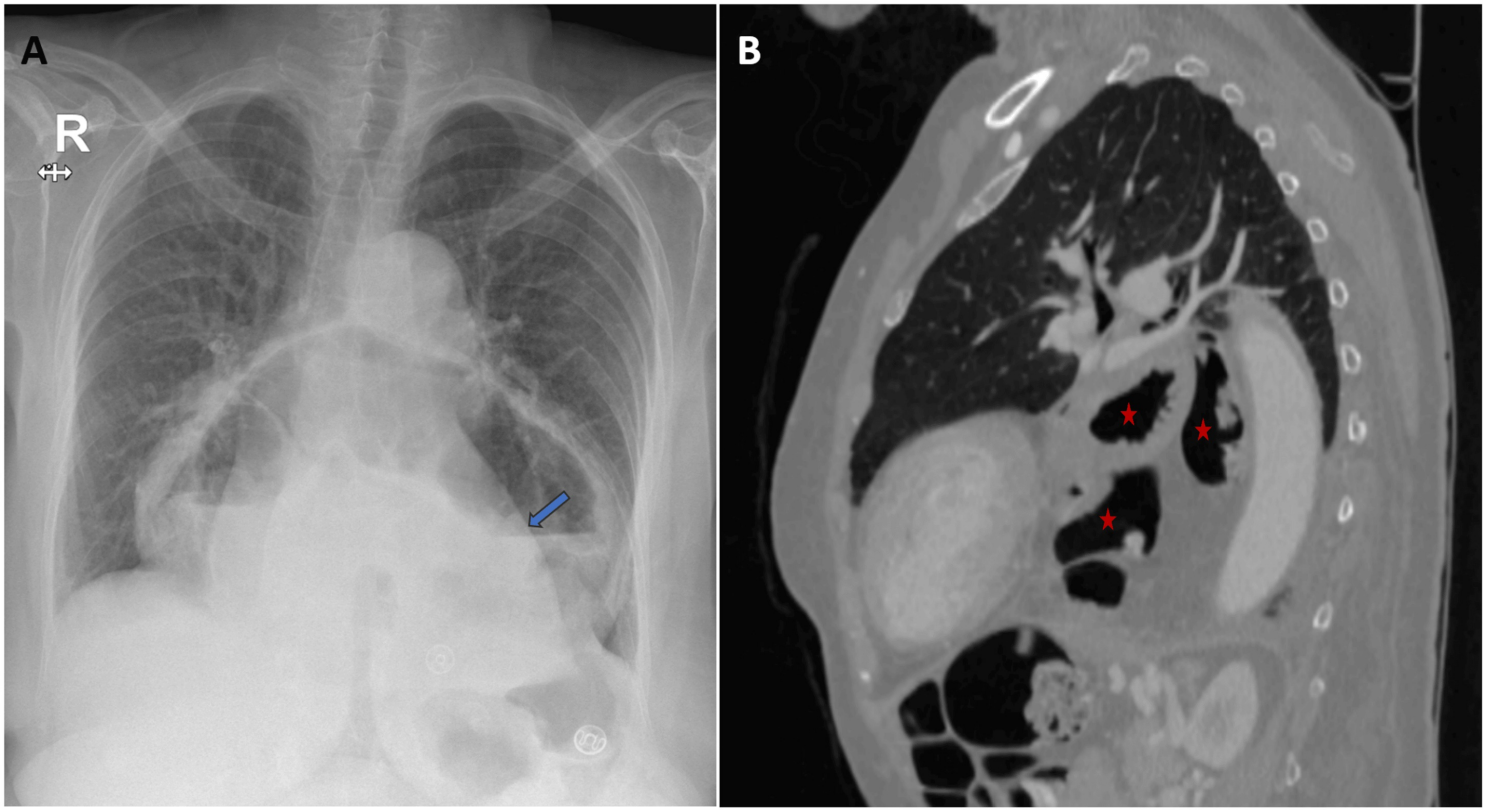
Left-sided diaphragmatic hernia Image A is a chest X-ray showing an air-fluid level overshadowing the cardiac silhouette (blue arrow). Image B is a sagittal CT image showing the presence of a left-sided diaphragmatic defect with the entire stomach, part of the transverse colon, and omental fat herniating into the thoracic cavity (red stars). CT: computed tomography
